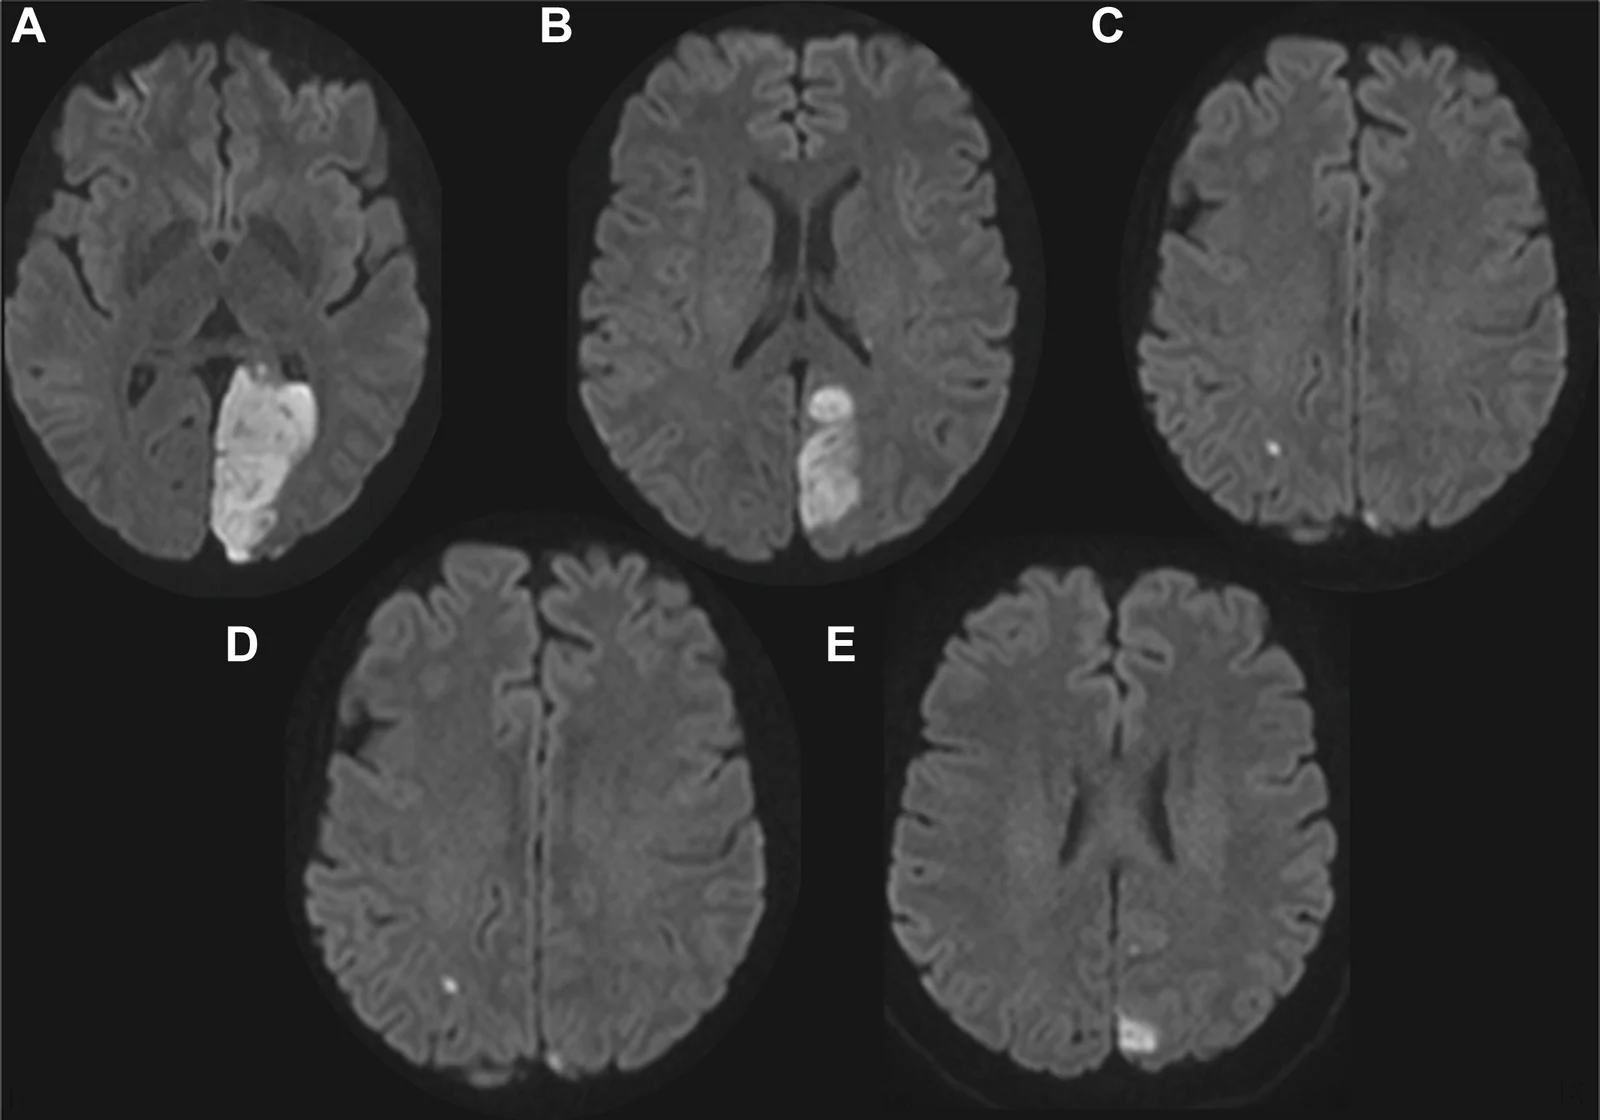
Brain Magnetic Resonance Imaging, Diffusion-Weighted Imaging Sequences (A and B) Large hyperintense area with restricted diffusion consistent with recent ischemic infarction in the left posterior cerebral artery territory, involving the left occipital cortex, subcortical region, and medial parietal hemisphere. (C, D, an E) Additional smaller foci with similar signal characteristics, consistent with recent ischemic lesions, are present in the left hemisphere at the posterior cingulate gyrus and along the lateral wall of the posterior left lateral ventricle. The presence of multiple recent bilateral ischemic lesions involving cortical territories supports an embolic etiology of the stroke.
